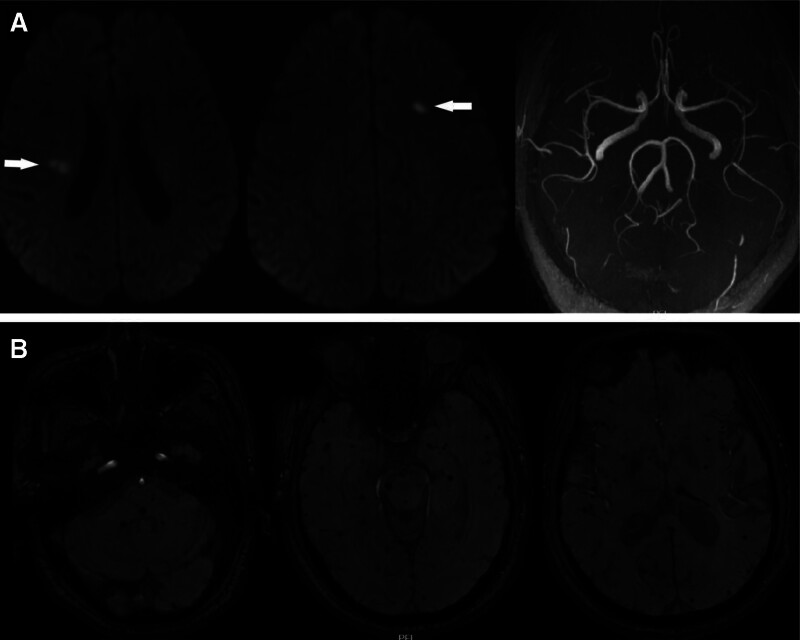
(A) DWI showed acute infarction foci in the right paraventricular body and left frontal lobe; MRA did not reveal any obvious abnormality. (B) SWI showed multiple microhemorrhagic foci/hemosiderin deposits in the brain parenchyma. DWI = diffusion-weighted imaging, MRA = magnetic resonance angiography, SWI = susceptibility-weighted imaging.
